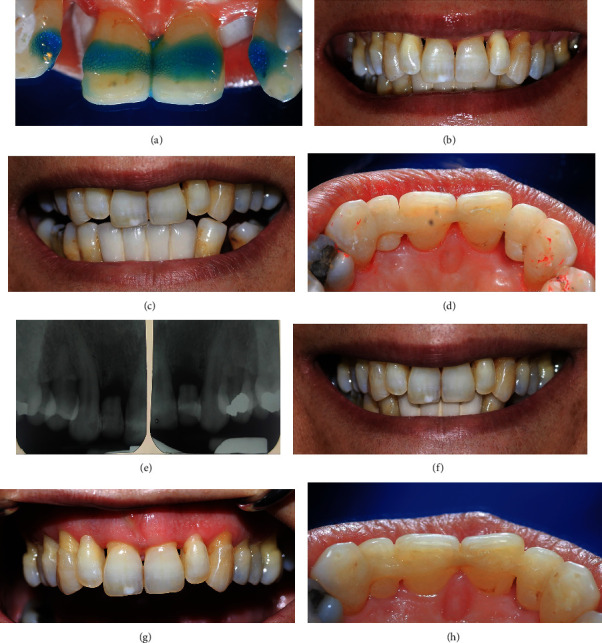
(a) Etching the prepared surface. (b) Close-up frontal view immediately after resin-bonded bridge (RBB) fabrication: reconstruction of natural smile and correction of crowding (c) occlusal contact in protrusive movement. (d) Palatal view before finishing and polishing. (e) Six-month follow-up: X-ray radiography. (f) Six-month follow-up: smile view. (g) Well adoption of pontics to the ridge six-month follow-up, there is no evidence of inflammation or recession in the soft tissue. (h) Palatal view of RBB after six months.
